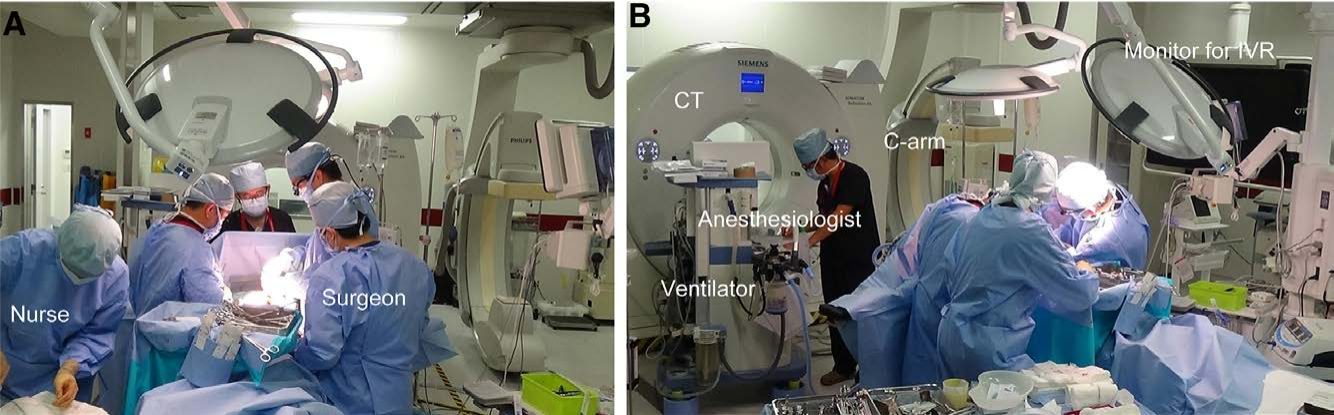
Hybrid emergency room system at the time of emergency surgery (A and B). CT = computed tomography, IVR = interventional radiology.
